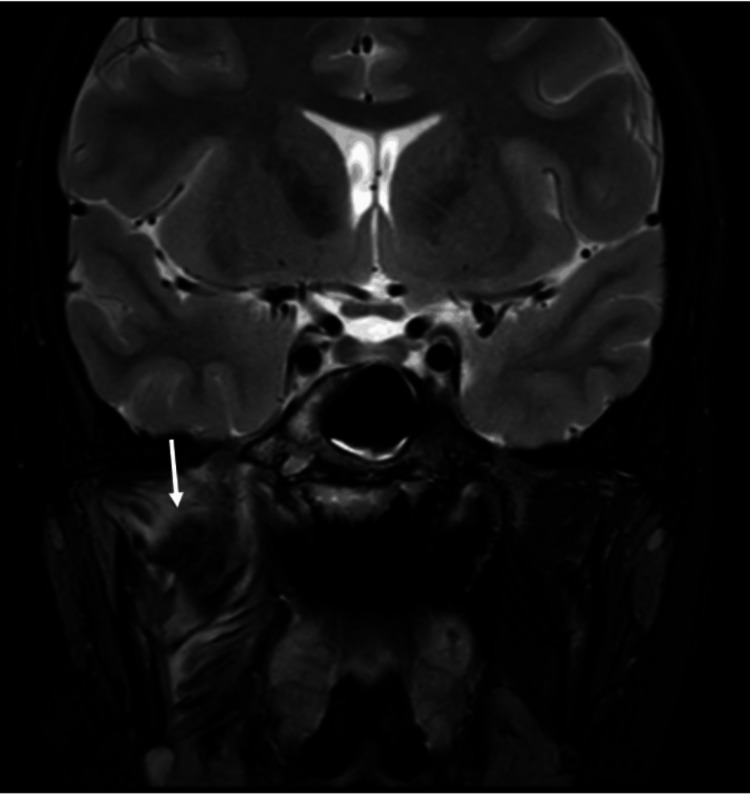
Third hospital admission. Coronal slice repeat MRI head and sinus with contrast showing significant inflammatory changes in the right masticator space but no identifiable collection and improvement of localised inflammatory changes of the dura and right TMJ
